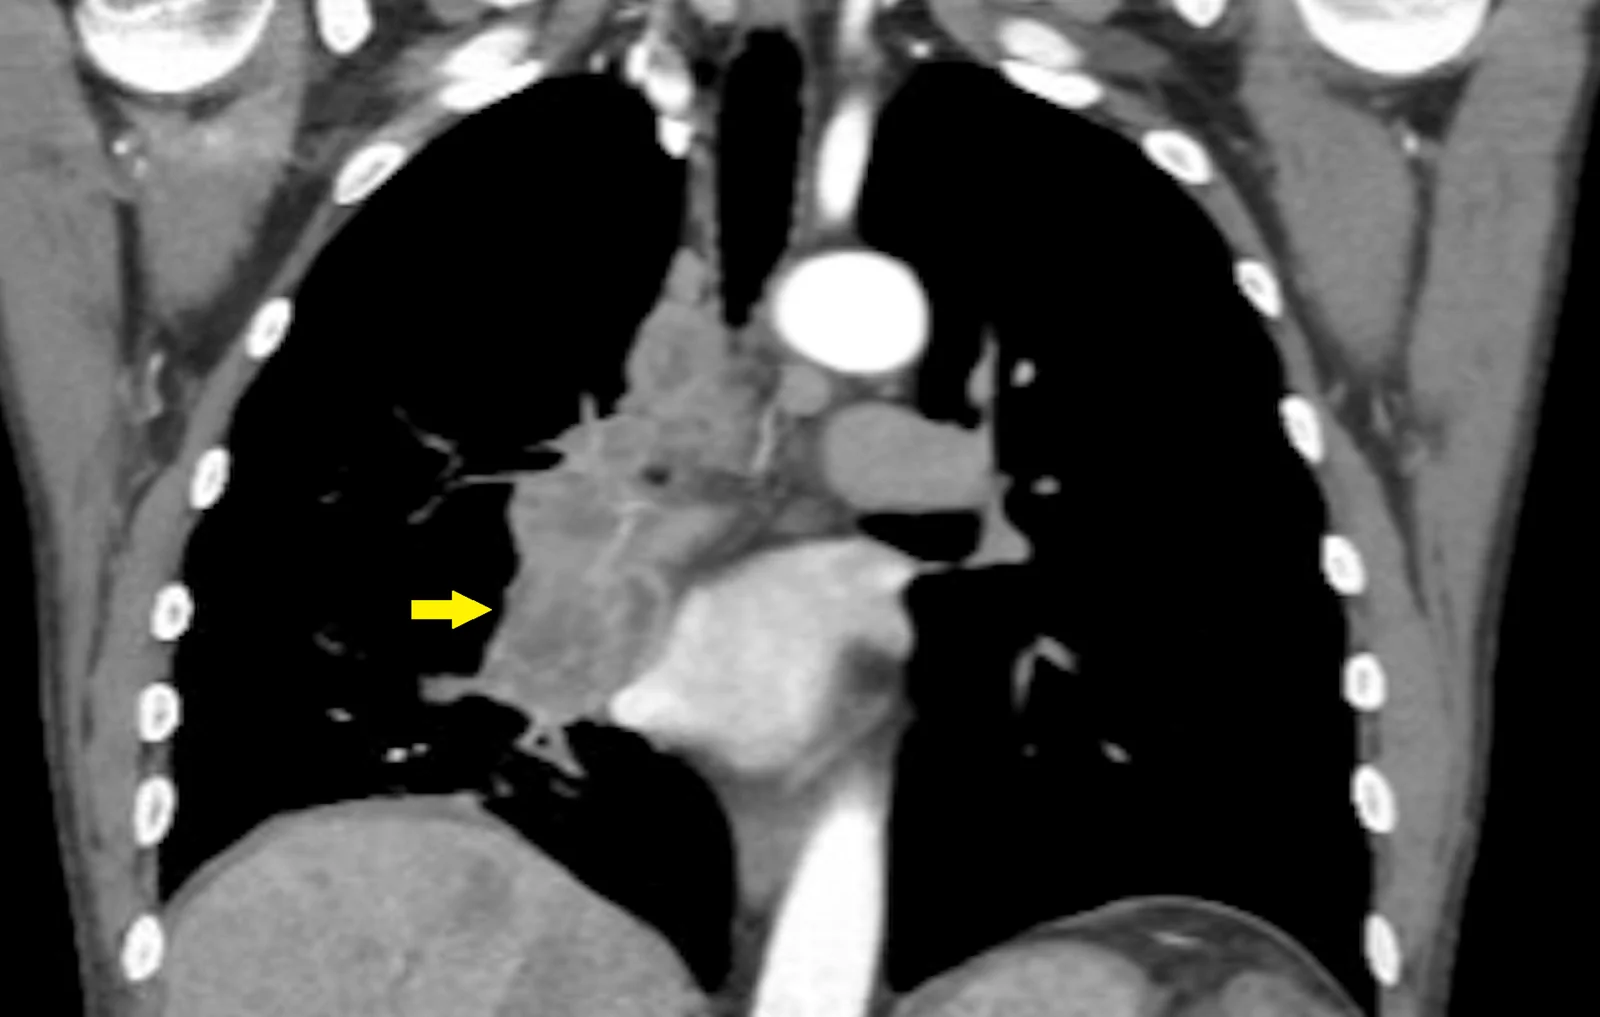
CT scan with IV contrast of the chest demonstrating a right perihilar mass The yellow arrow is pointing towards the 4.7 x 3.1 cm cystic and necrotic right perihilar mass.
